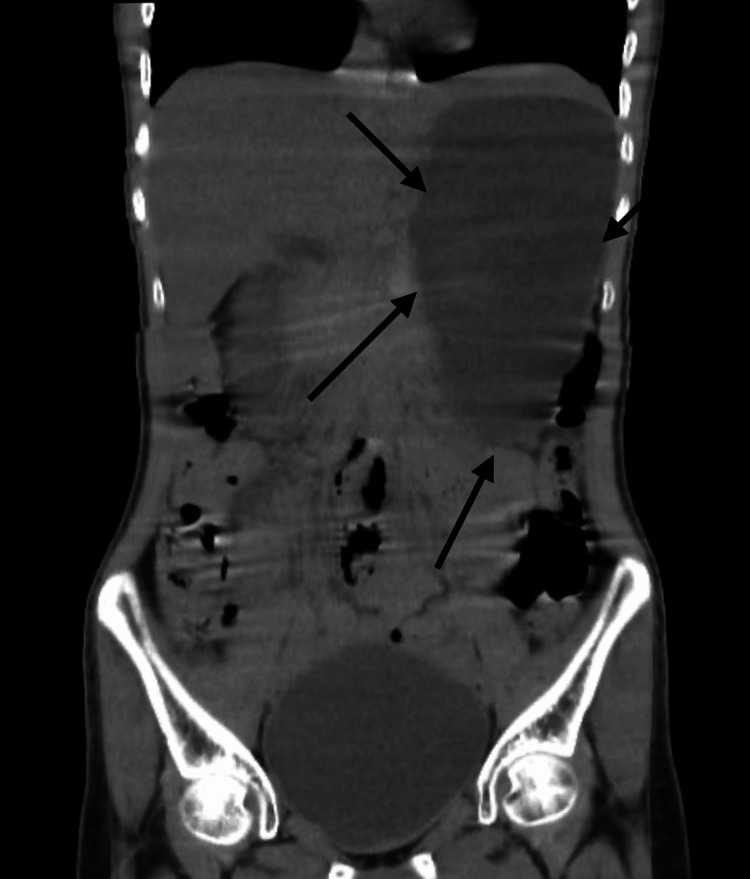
Abdominal CT at 10 weeks after initial presentation showing marked gastric distension The arrows indicate the markedly distended, fluid-filled stomach. An air–fluid level was present in the antral projection, with marked, predominantly hypodense distension of the duodenum (first and second portions); there was no pneumoperitoneum or free fluid. CT, computed tomography.
